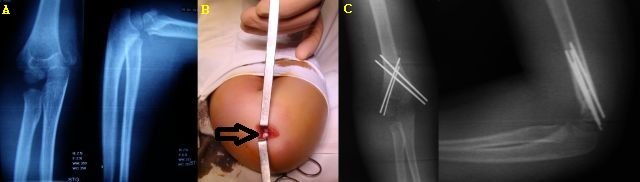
Supracondylar humeral fracture that was reduced and stabilized by using a minimally invasive technique. B. The ulnar nerve is identified through a minimal incision, in order to avoid injuring it. C. Postoperative X-ray.
